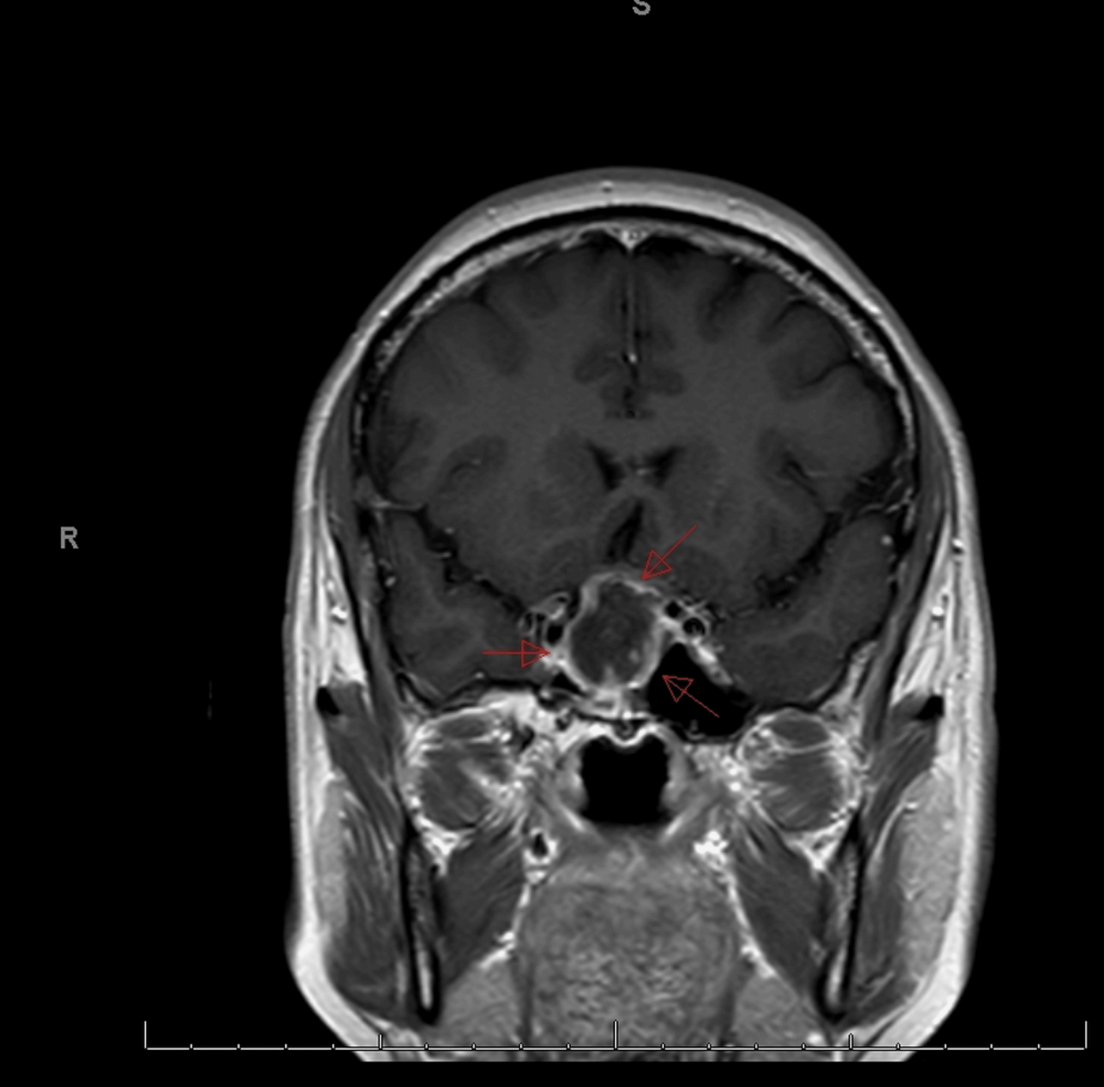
Contrast-enhanced T1-weighted (T1W) coronal magnetic resonance imaging (MRI) The peripheral enhancement of the lesion, which is eccentric to the right side, is well visualized in the coronal sequences.
